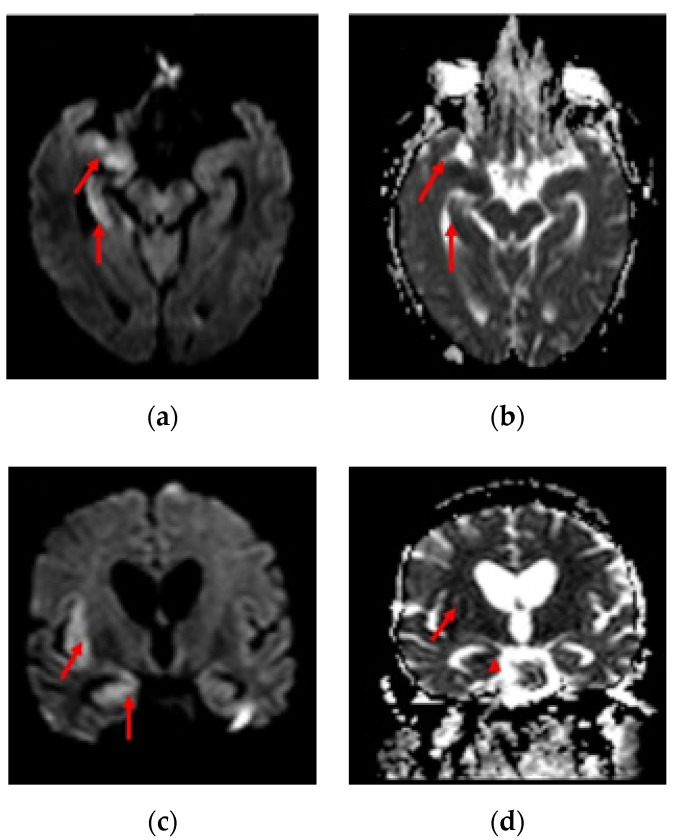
54-year-old man with COVID-19 RT-PCR positive and history of fever, cough for 7 days with recent onset headache and vomiting. MRI DWI (a) and ADC (b) showed diffusion restriction with corresponding hypointensity on ADC (red arrow) in the medial aspect of right anterior temporal lobe, hippocampus and sylvian cortex. MRI DWI (c) and ADC (d) showed diffusion restriction with corresponding hypointensity on ADC (red arrow) in the medial aspect of right anterior temporal lobe, hippocampus and sylvian cortex.
